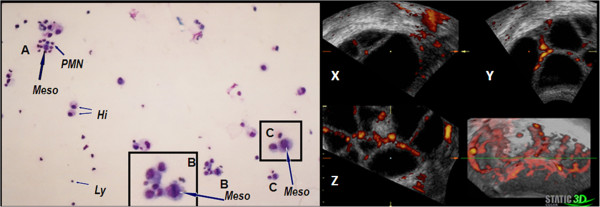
Cytologic examination of cells in the ascitic fluid of a patient with ovarian hyperstimulation and ultrasonographic assessment of OHSS. On the left side (A,C) atypical cells can be observed among the leukocytes (Ly), red blood cells, mesothelial cells (Meso) and histiocytes (Hi). Abnormal cells contain a dark cytoplasm that, in some places, resembles a seal-ring as well as large and rough-grained nuclei with multiple nucleoli. Mitosis and polymorphonuclear (PMN) epithelial-like cells can be seen in the smear (B; magnification 200X). The cytologic diagnosis was P IV: strong suspicion of an epithelial-like, malignant ovarian tumour. On the right side, three-dimensional (X,Y,Z; three orthogonal planes) Power Doppler ultrasonographic visualization of the ovaries in a case of severe OHSS that demonstrates ominous signs of hypervascularization (static 3D image).
